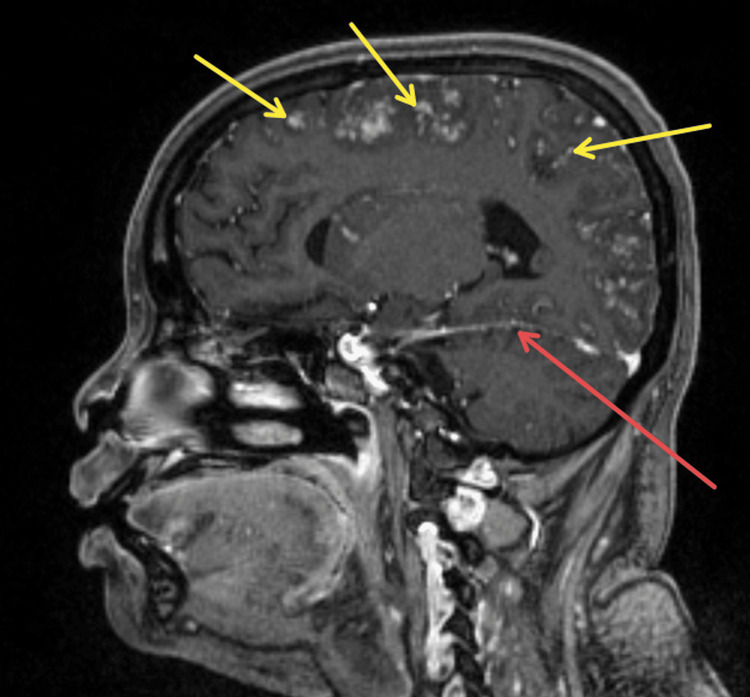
Sagittal post-contrast T1-weighted MRI demonstrating multiple nodular enhancing hyperintensities (yellow arrows), along with leptomeningeal enhancement (red arrow).
